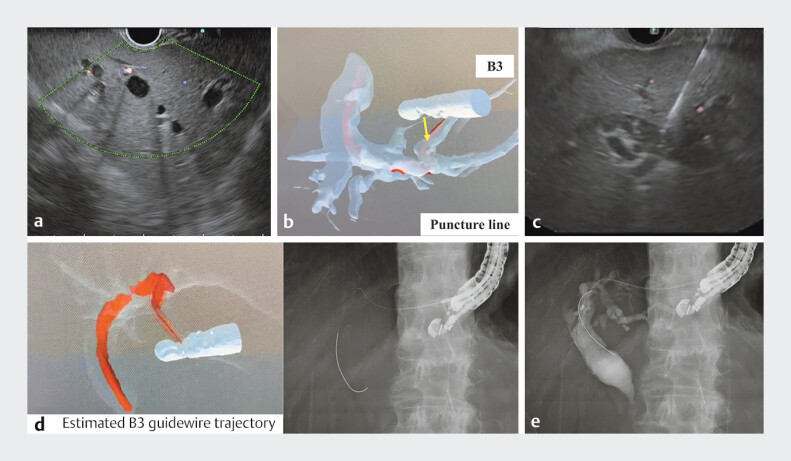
Endoscopic ultrasound-guided hepaticogastrostomy (EUS-HGS) using spatial reality display (SRD) navigation capable of naked-eye stereopsis.
